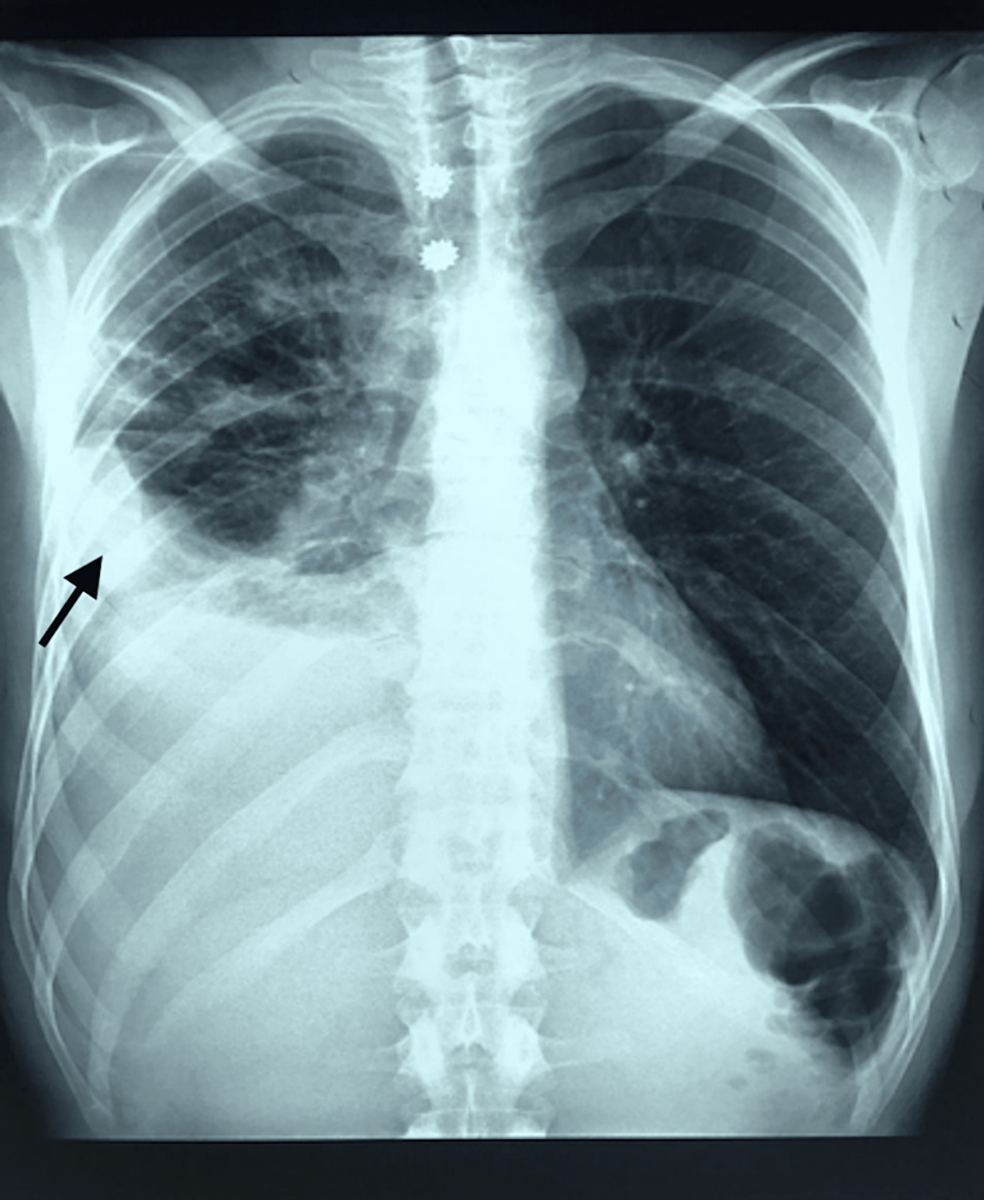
Chest X-ray showing right unilateral pleural effusion (black arrow)
